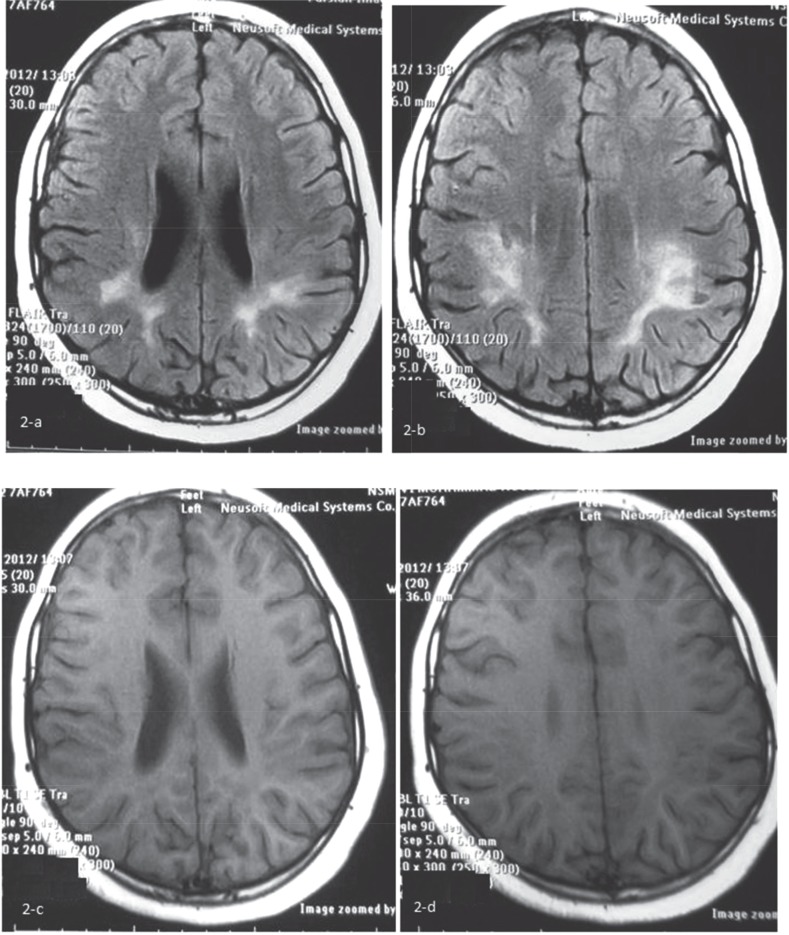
Brain MRI with contrast showed remaining hyper signal in T2- FLARE from sequel of treated demyelinating lesions (a,b) and just disappearance of tumefactive lesions in T1-WI (c,d)
